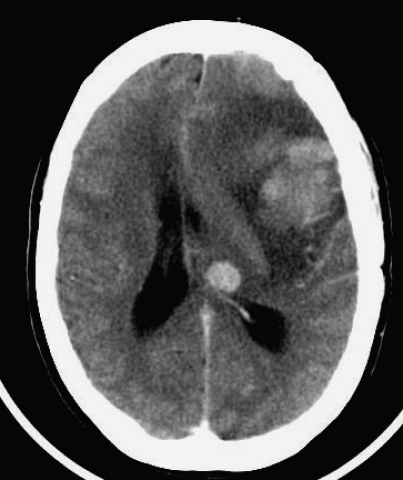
Post operative CT with intracranial haemorrhage and recurrence of the tumour.
